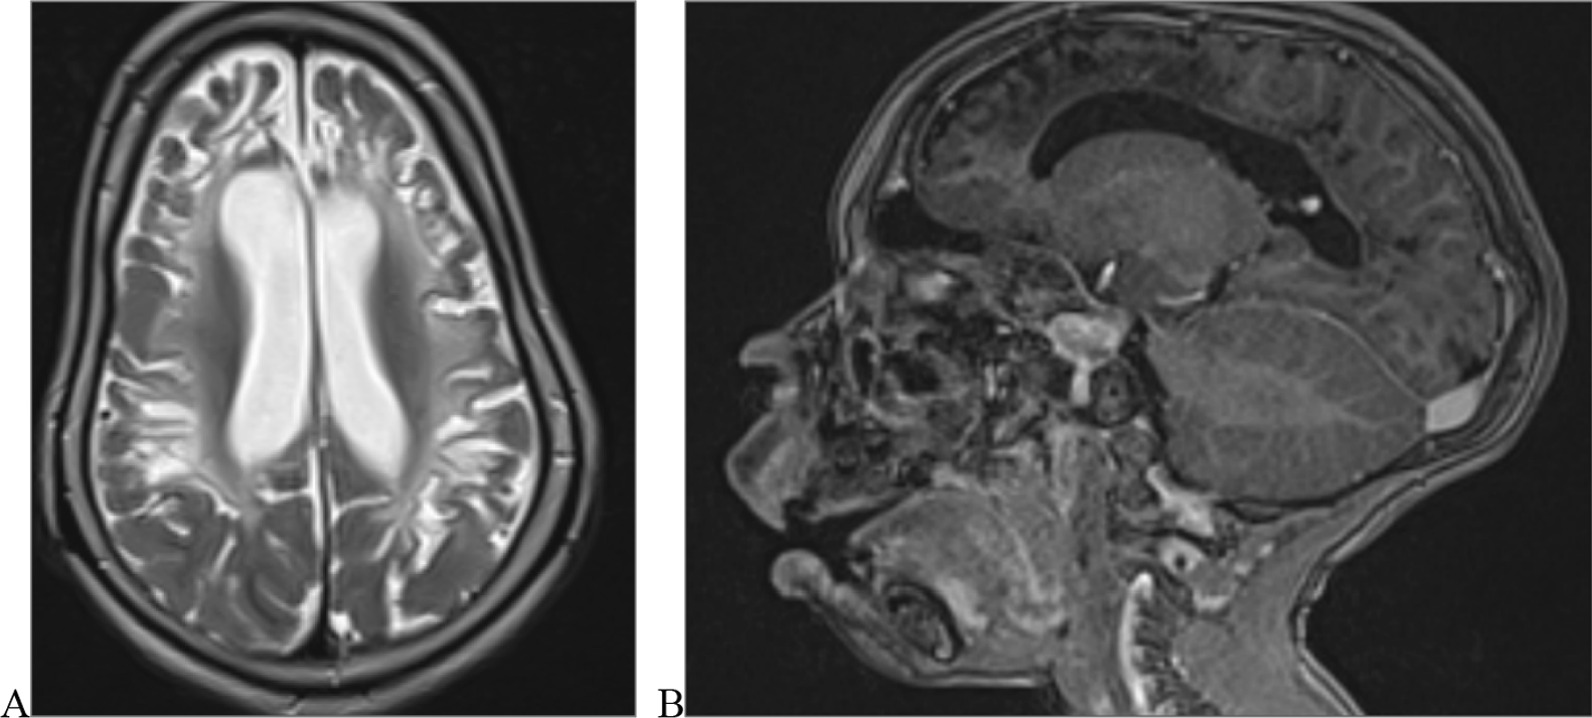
Seven year old male with epilepsy and developmental delay.Axial T2 weighted brain MRI (A) and sagittal MPRAGE (B) showing bilateral extensive fronto-parietal multicystic encephalomalacia with mushroom shaped gyri(ulegyria) in an infant who was born at term in a case of severe chronic ischaemic insult(hypoxic ischaemic encephalopathy)
